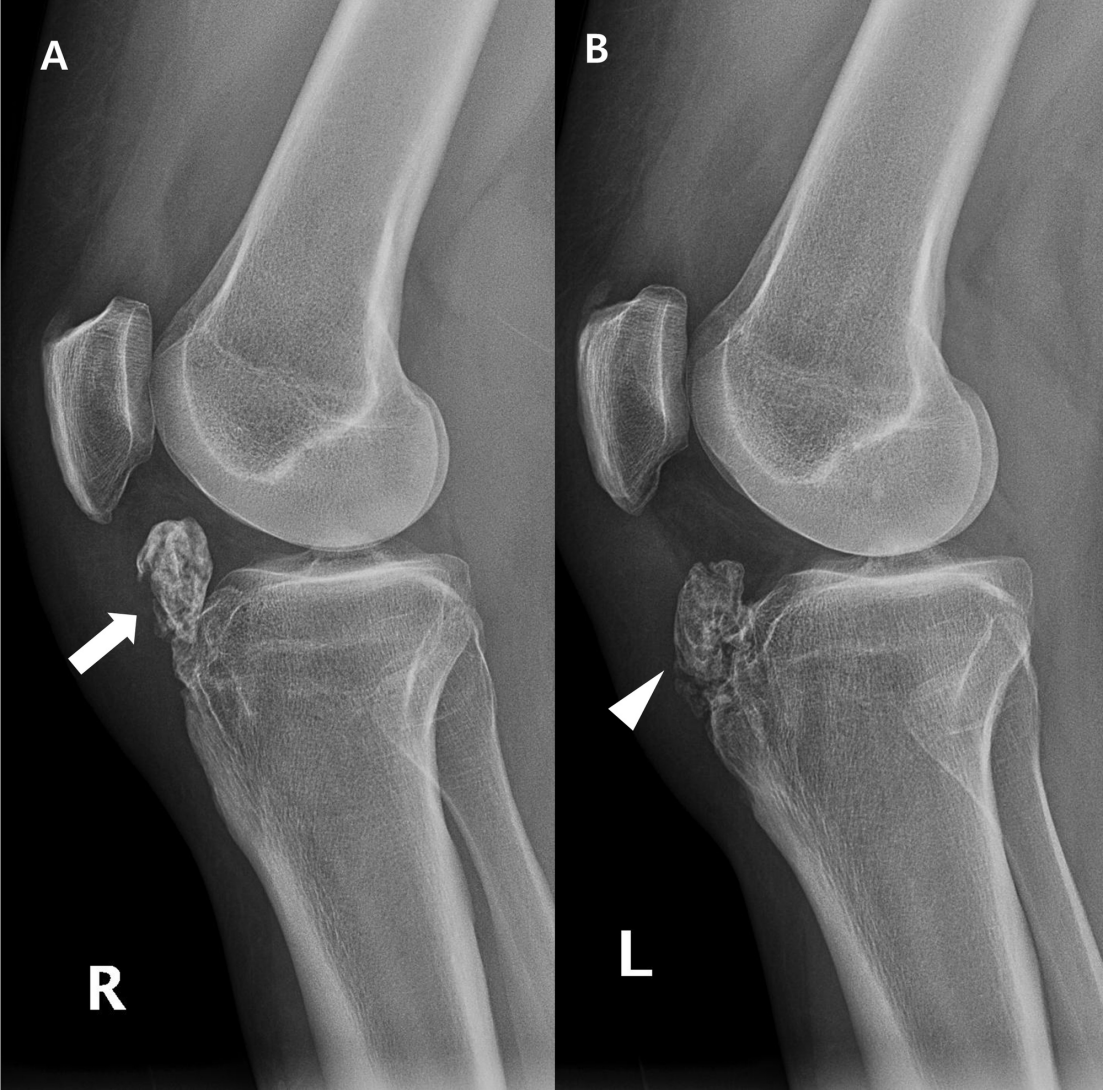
Lateral plain radiographs of both knees. (A) A large osseous lesion (arrow) is seen at the infrapatellar area of the right knee. (B) An osseous body (arrowhead) of equivalent size was partially fused to the hypertrophied tibial tuberosity of the left knee.
